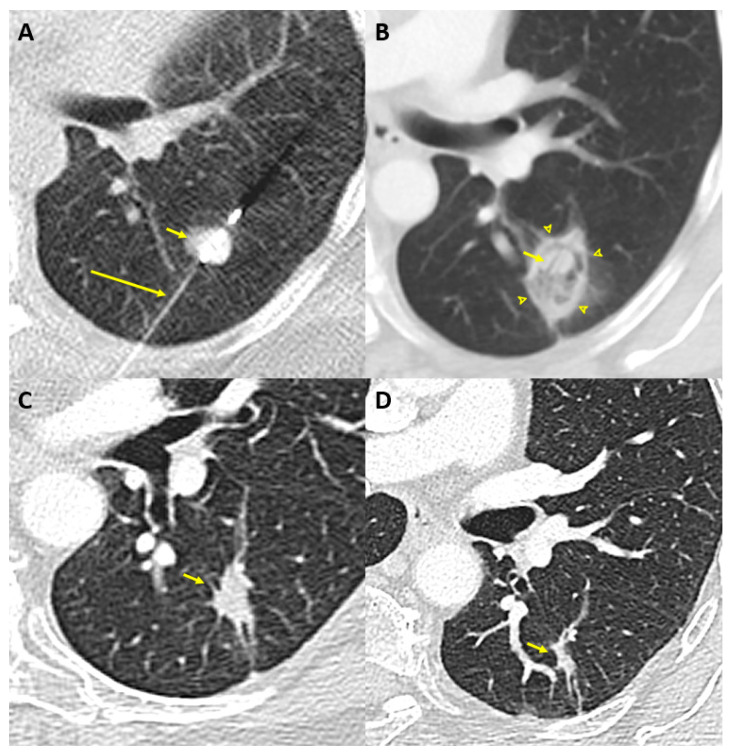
Example of MWA in a sixty-year-old woman with a 13 mm nodule in the apical segment of the left lower lobe. (A) Axial non-contrast chest computed tomography scan obtained during the MWA procedure shows the MWA probe (long arrow) placed percutaneously in the nodule (short arrow). (B) The computed tomography scan performed 6 weeks post-ablation shows a ground glass opacity (arrowheads) surrounding the nodule (short arrow) and corresponding to the ablation zone. In this case, technical success was confirmed with an ablation margin above 10mm. (C) Chest-computed tomography performed 13 months later confirmed the expected decrease in size post-ablation (short arrow). (D) Sixty-one months later, the post-ablation zone markedly decreased in size (short arrow), with no signs of local recurrence.
